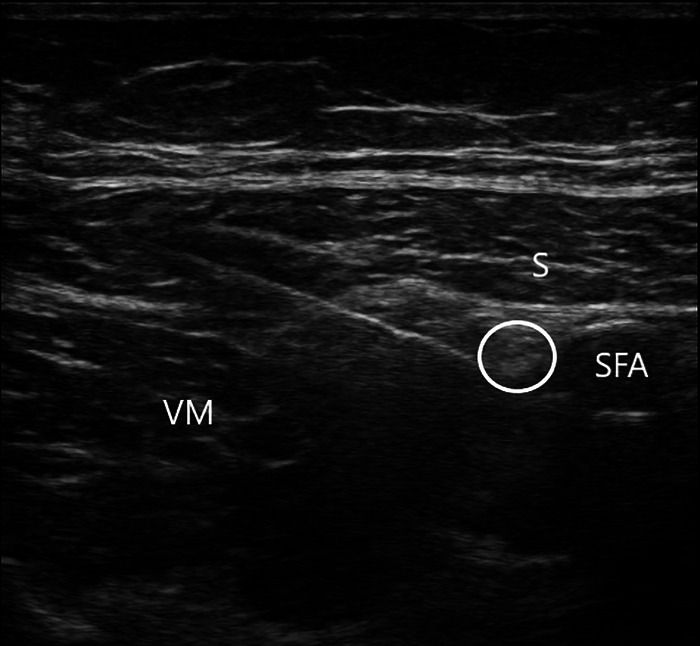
In this image of an ultrasound-guided adductor canal block, the needle can be seen entering from the lateral side between the sartorius (S) and the vastus medialis (VM) muscles. The target is the circled saphenous nerve that is just lateral to the superficial femoral artery (SFA).
